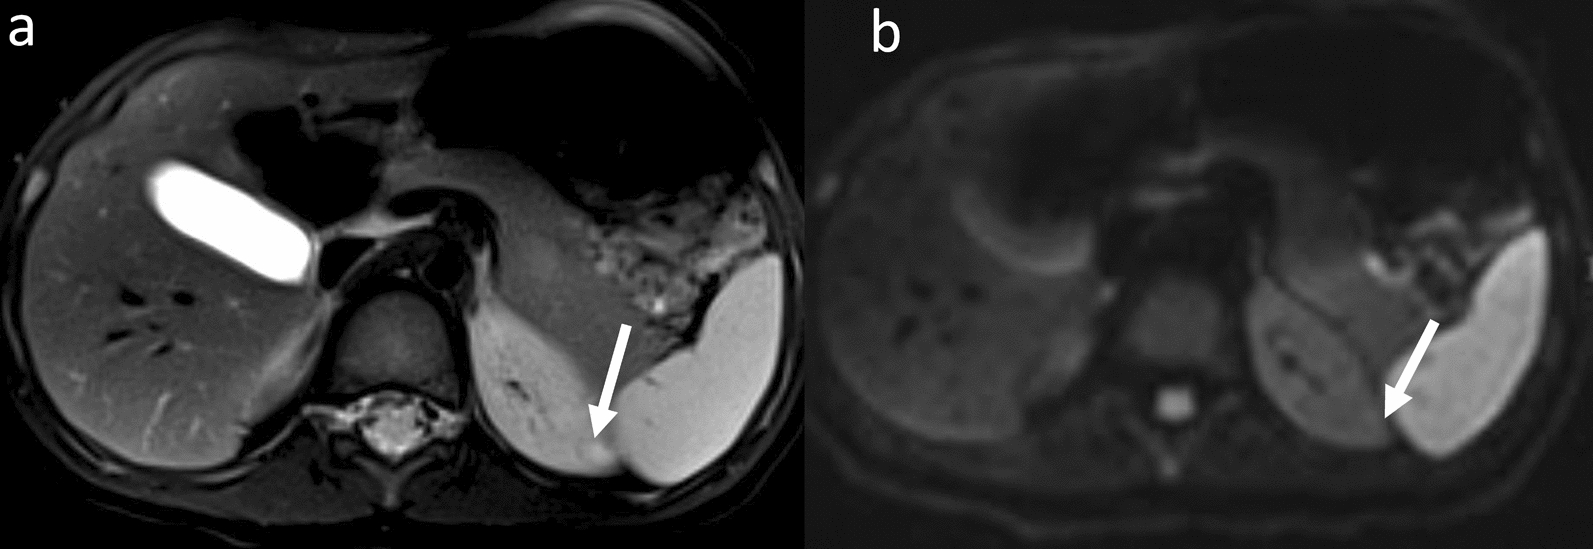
MRI of a simple cortical cyst in a 2 year, 9-month-old boy with BWS^IC2^. Arrows indicated a subtle hyperintense signal in the left kidney on the fat-suppressed T2WI (a). The lesion was invisible on DWI (b) and showed no diffusion restriction
